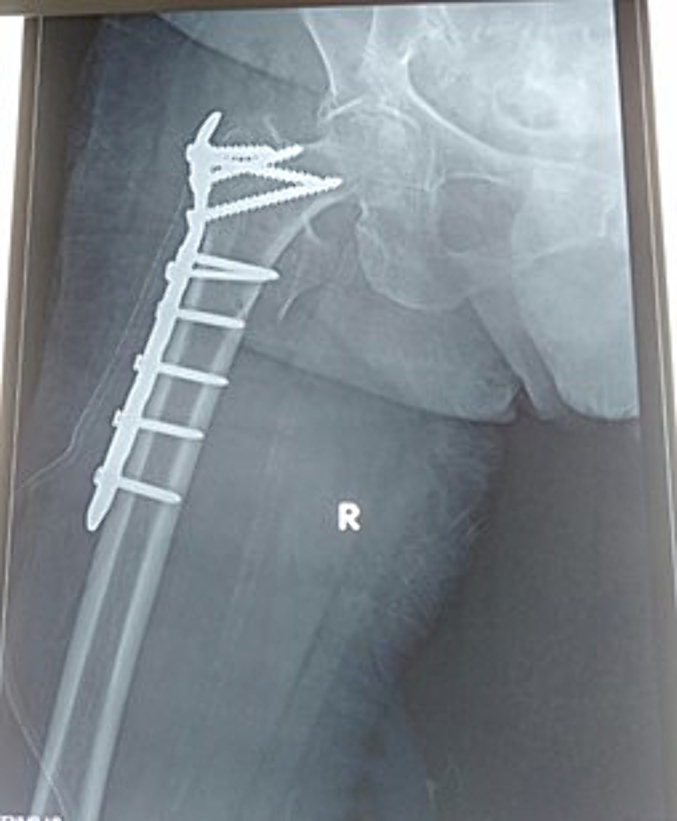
Plain Xray of post intertrochanteric plating with interlocking plate.
